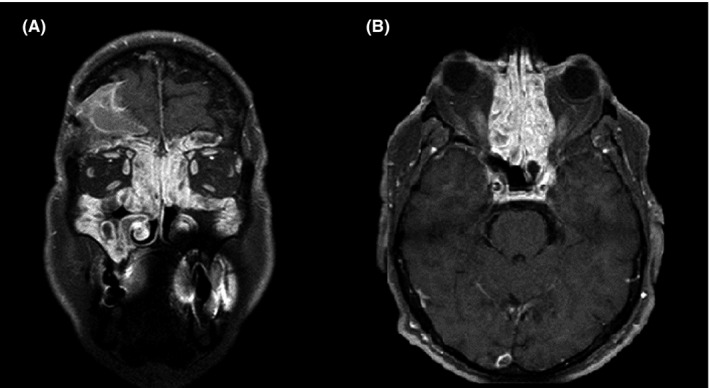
Coronal (A) and axial (B) CT scan performed before dupilumab treatment. Imaging shows a massive recurrence of nasal polyposis resulting in complete obstruction of the maxillary, frontal, and ethmoidal paranasal sinuses
